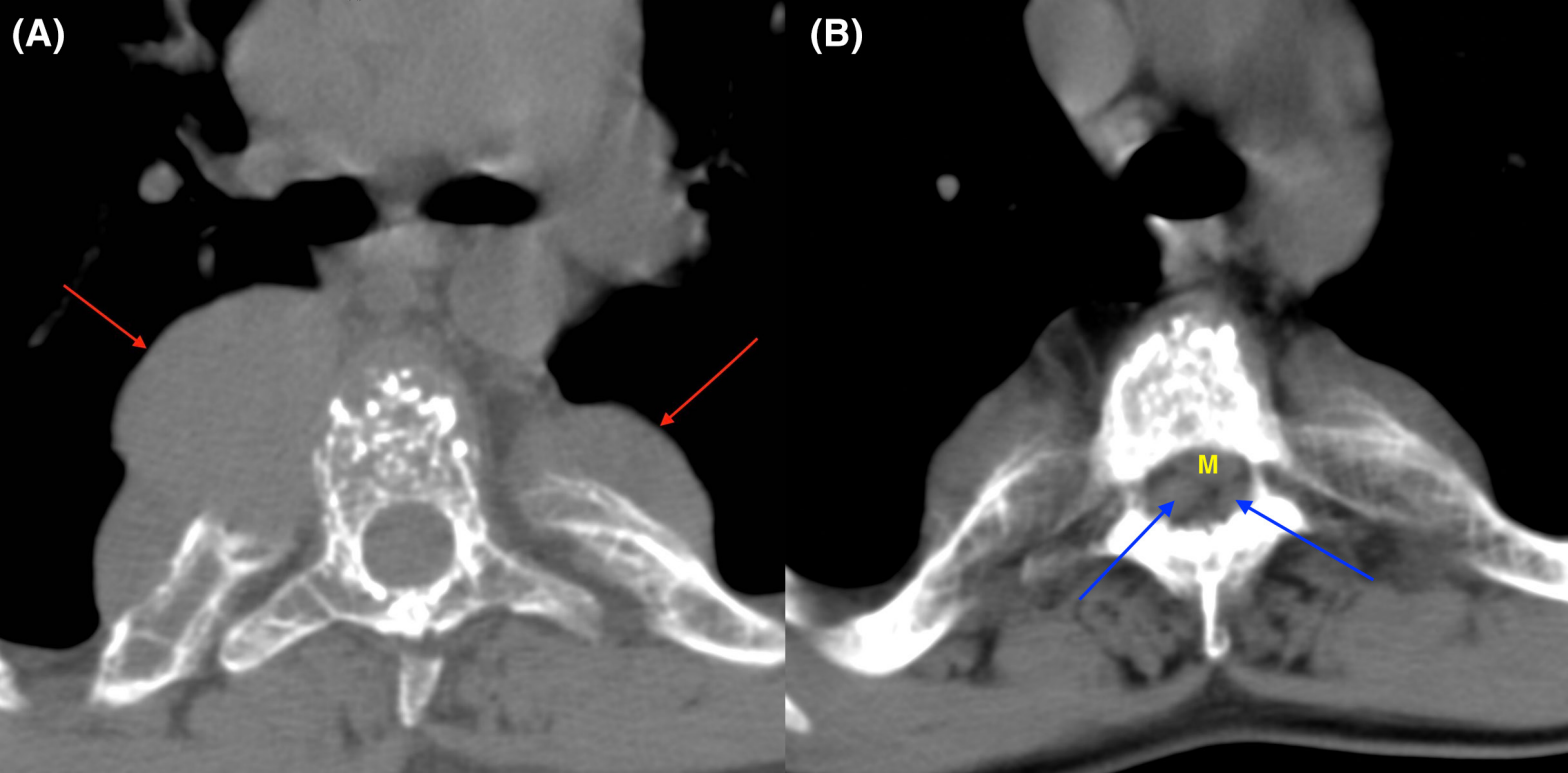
Spine CT: (A) thoracic paravertebral lesions labeled with red arrows; (B) lesions inside the spinal canal at the thoracic level, labeled with blue arrows, narrowing the spinal canal and compressing the medulla (M).
